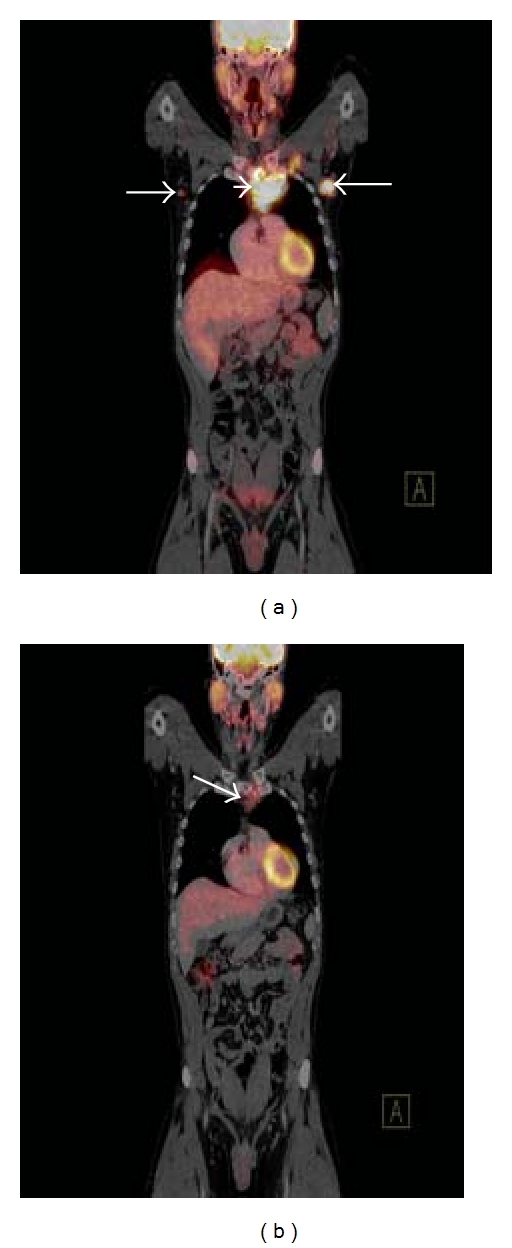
Coronal fused FDG-PET/CT image (a) of a patient with nodular sclerosis type Hodgkin's lymphoma prechemotherapy demonstrating an FDG-avid lymph node mass in the superior mediastinum (white arrowhead) and hypermetabolic bilateral axillary lymphadenopathy (white arrows). Interim staging coronal fused FDG-PET/CT image (b) in the same patient after 3 cycles of chemotherapy demonstrating a significant interval decrease in both the degree of FDG uptake and the size of the lymph node mass in the superior mediastinum (white arrow) with resolution of the axillary lymphadenopathy.
